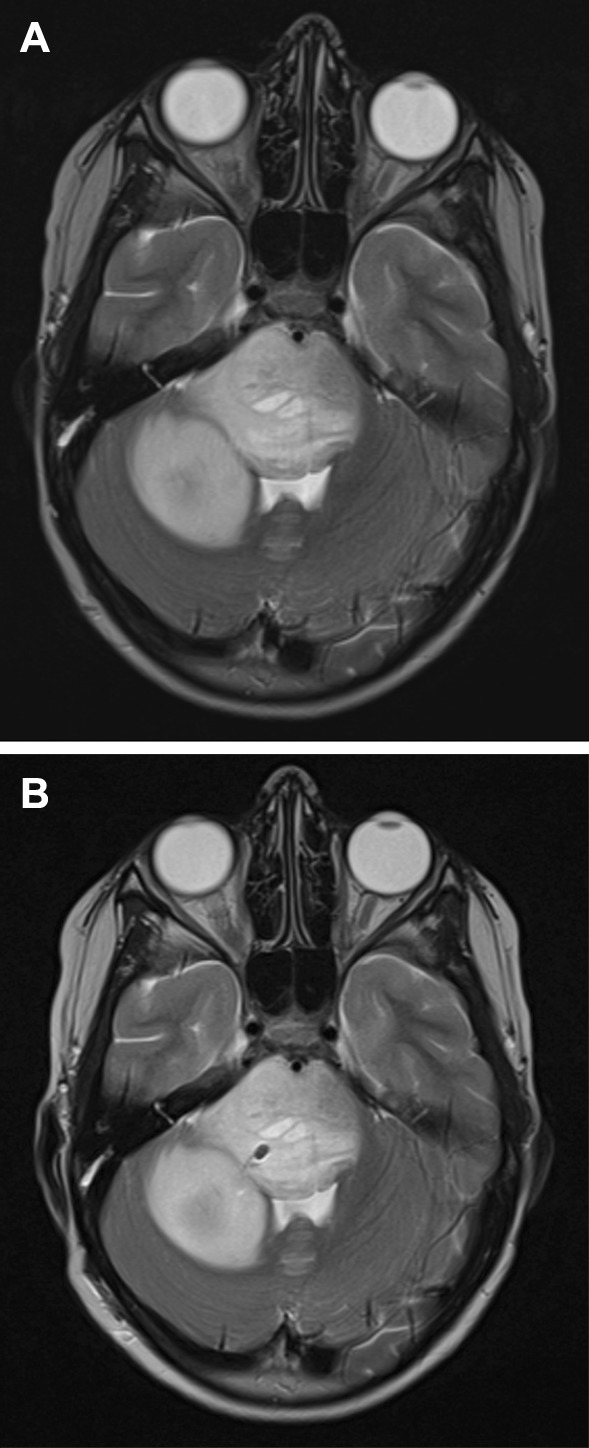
a Pre-operative and b post-operative axial T2-weighted MRI scan of demonstrating pontine lesion and right-sided transcerebellar approach
